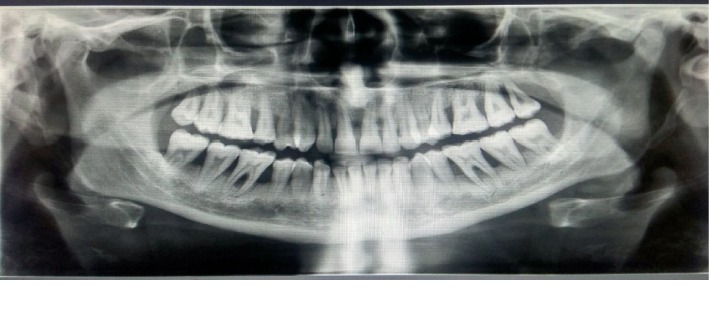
Panoramic radiograph showing extensive generalized periodontal breakdown.
